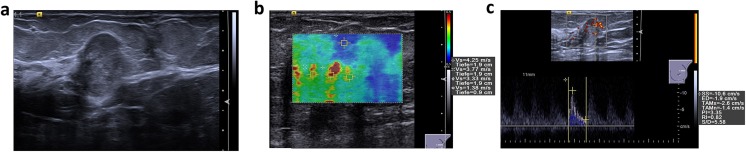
Multiparametric ultrasound of a palpable, 17-mm large lesion in the craniolateral left breast of a 70-year-old patient. a In B-mode, the lesion was isoechoic, slightly heterogeneous, with a partially indistinct margin and an anti-parallel orientation and was classified as BI-RADS 4c. b In VTIQ elastography, the lesion demonstrated hard parts, with a SWVmax of 4.25 m/s. c Power Doppler showed abundant internal vascularity and the spectrum analysis of the most prominent artery demonstrated an RI of 0.82. The application of the classification algorithm would classify this lesion as node 2, with a very high probability of malignancy. Ultrasound guided biopsy revealed a Grade 1 mucinous carcinoma
